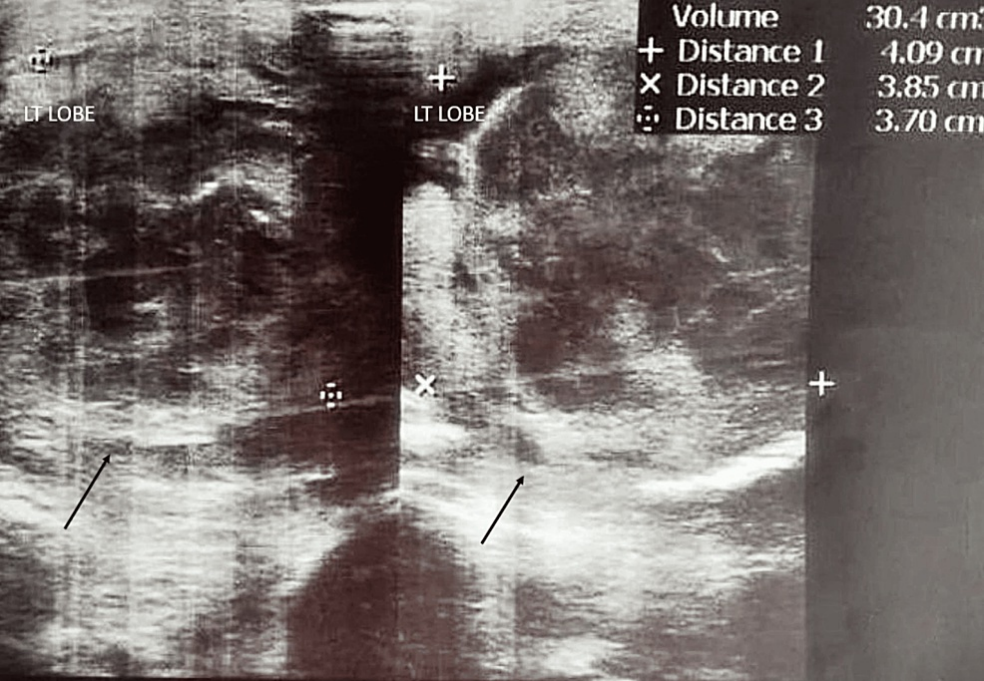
Ultrasound image showing a large heteroechoic mass in the left lobe of the thyroid containing fluid and echogenic contents, measuring 40 x 38 x 37 mm (shown in black arrow)
